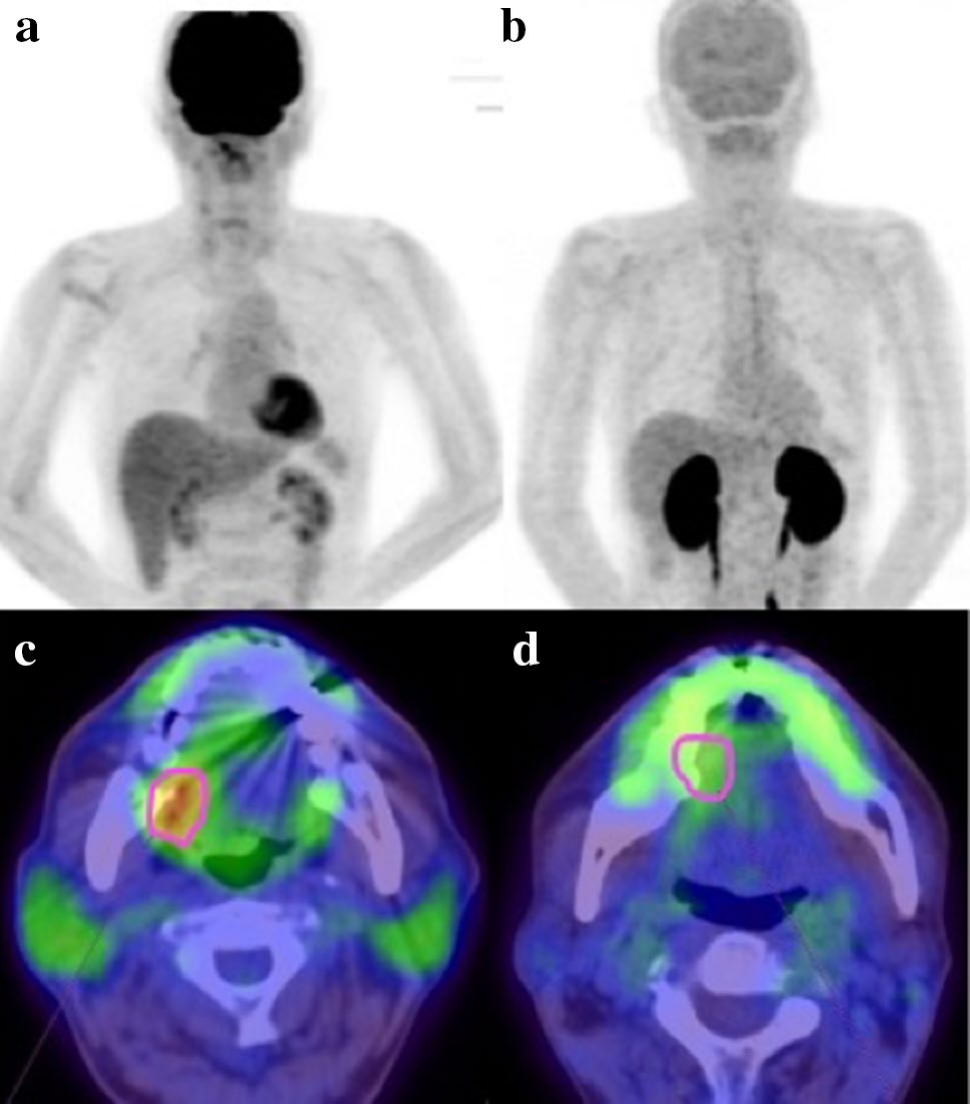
Maximum intensity projection imaging of a patient with a poor outcome using a^18^F-FDG, b^18^F-FAMT, and primary PET/CT imaging by c^18^F-FDG and d^18^F-FAMT
